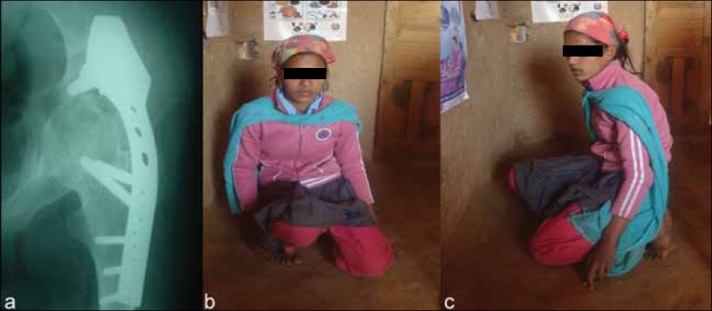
(a) Anteroposterior X-ray of left hip at 9.5 years follow up showing cobra plate *in situ* and successful fusion. (b,c) Clinical photograph at 9.5 years of same patient showing good function
